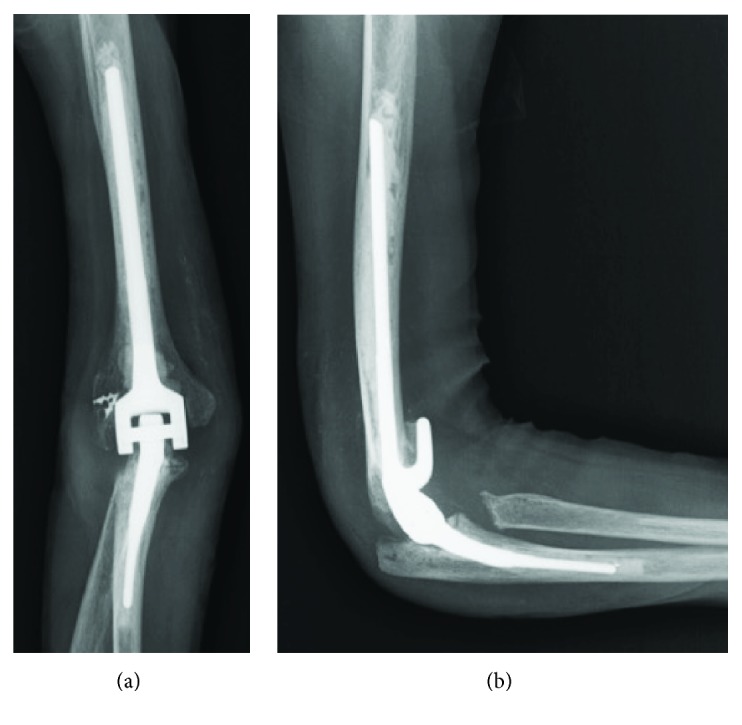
(a) AP and (b) lateral radiographs of the right elbow following a long-stemmed cemented Coonrad-Morrey TEA.
